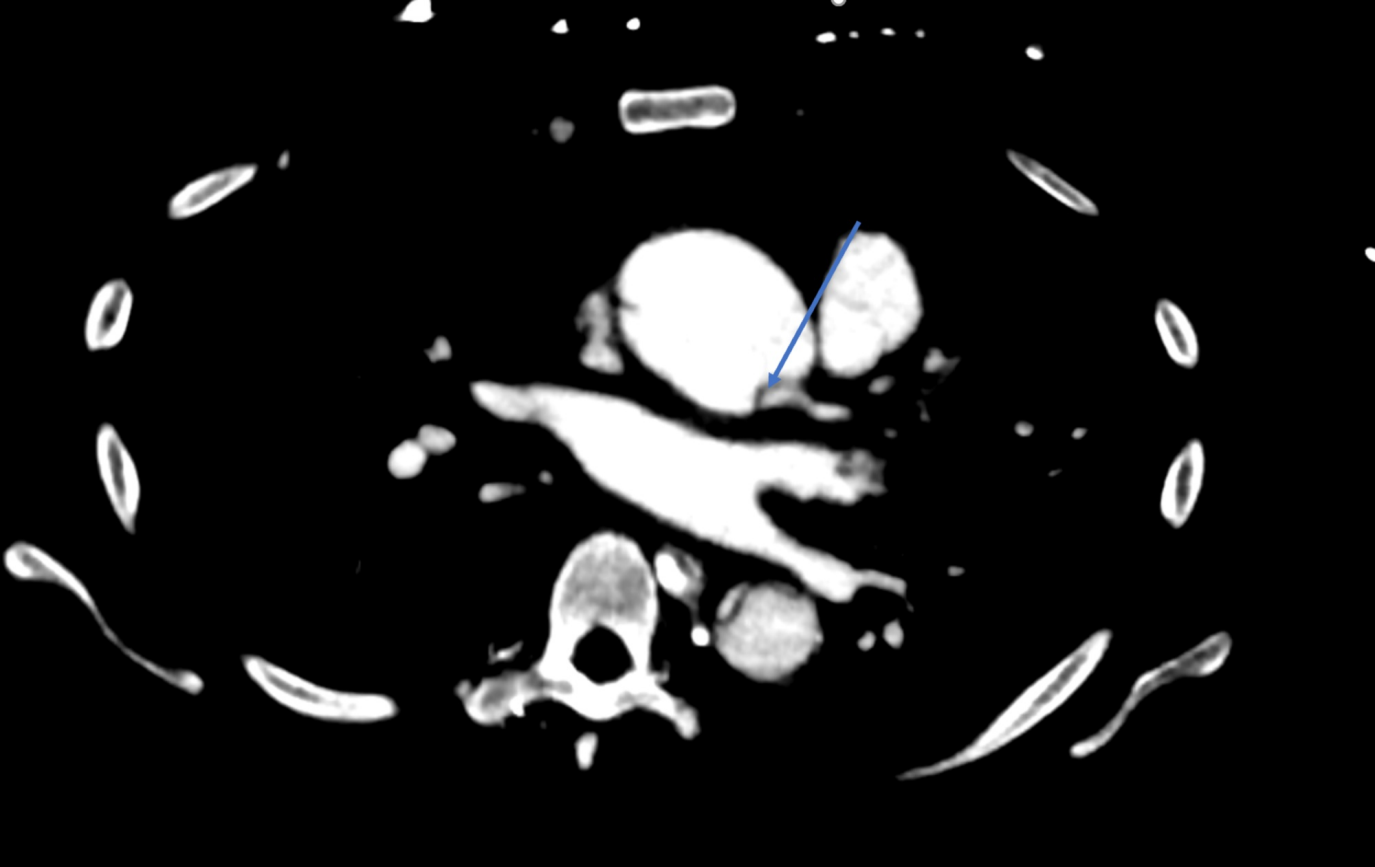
CT scan showing extension of dissection to the coronaries
